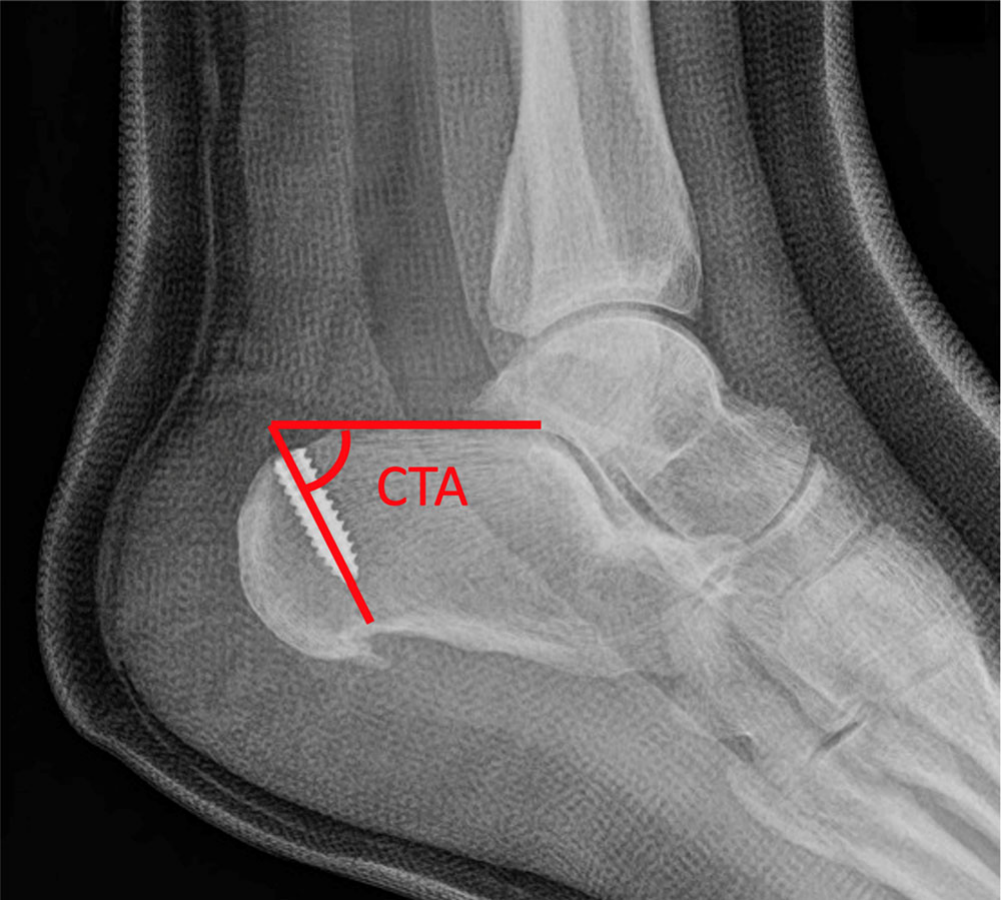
The calcaneal tunnel angle (CTA).
